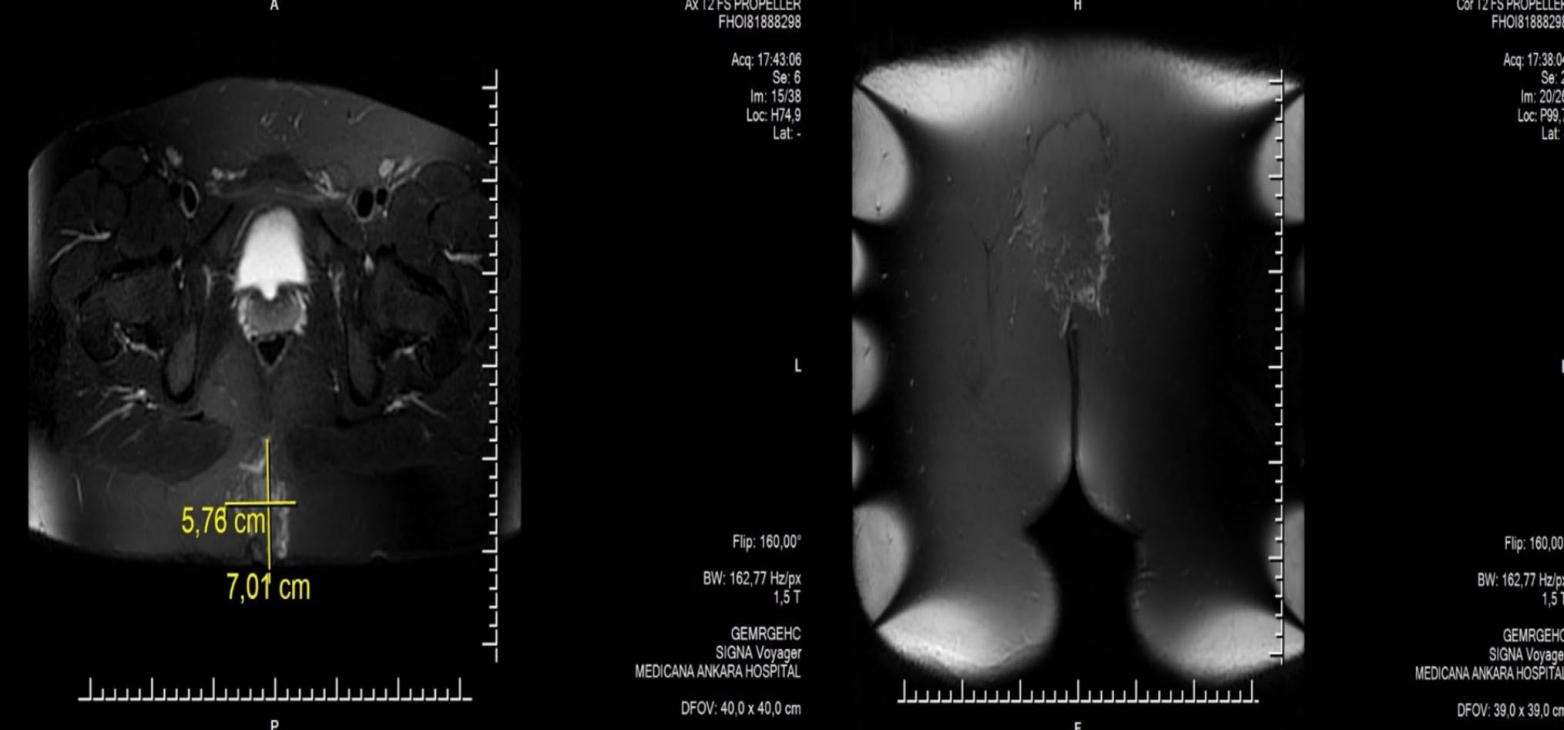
Preoperative MRI image of the patient who had previously undergone Limberg flap due to pilonidal sinus disease
